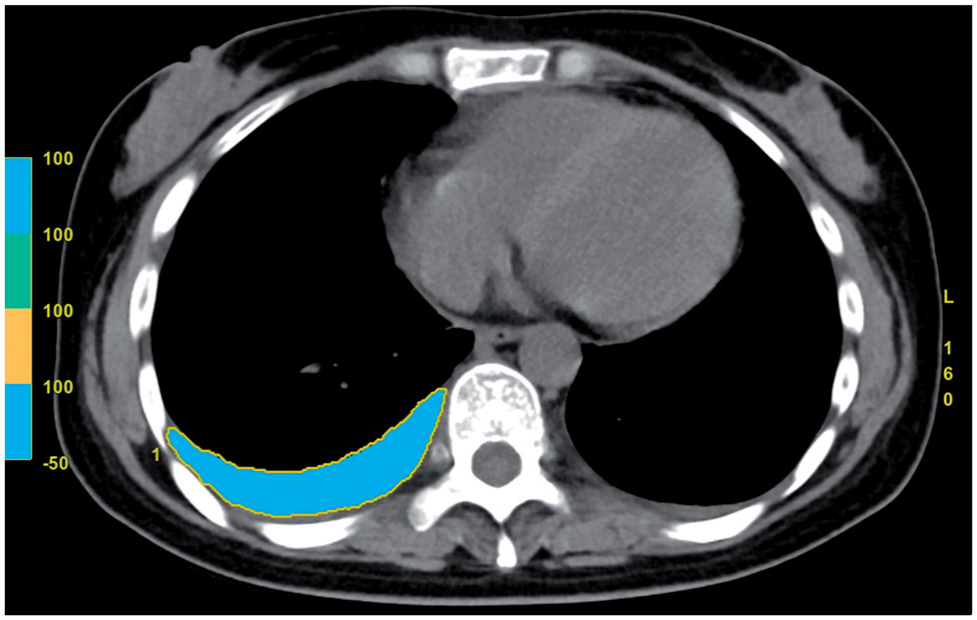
The measurement of pleural effusion volume with chest CT images.
